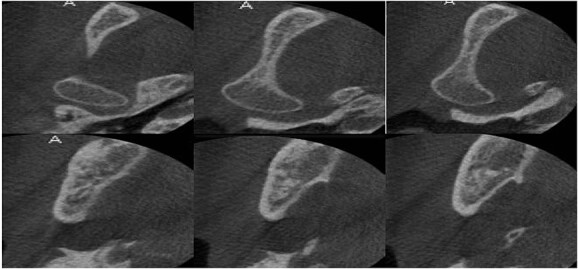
Cone beam computed tomography after 30 months showed full healing without any recurrence.
